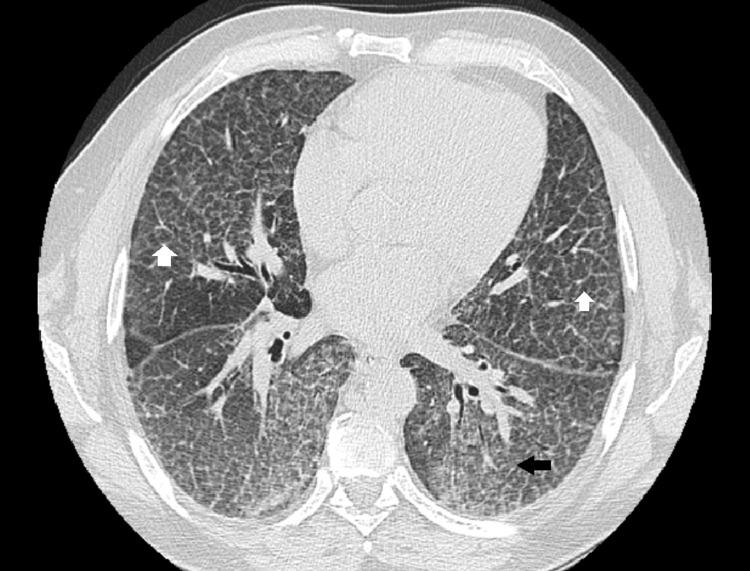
Thoracic CT scan showing ground-glass opacities (black arrow) with interlobular septal thickening (white arrows).
